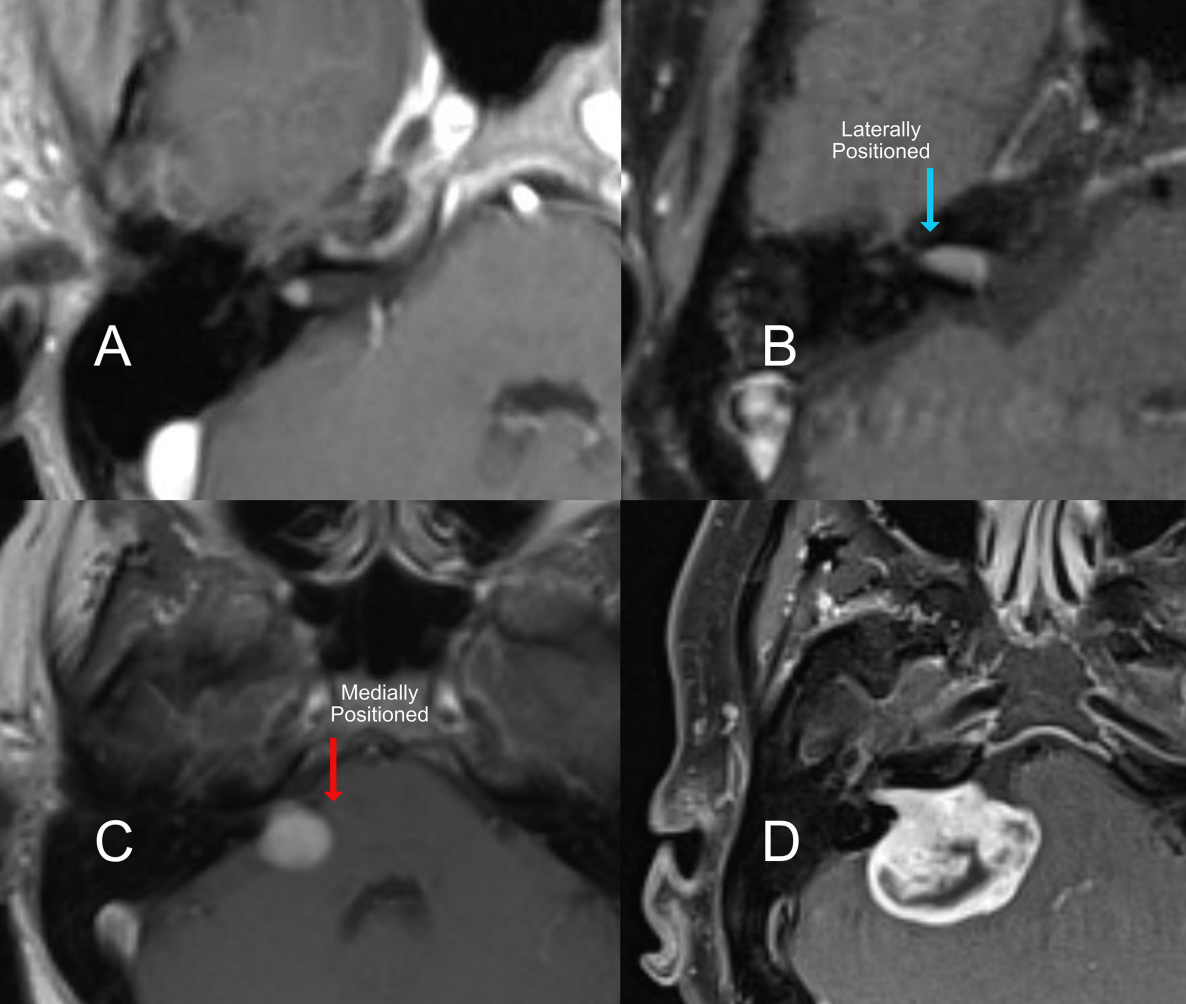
T1 Contrast MRI of an acoustic neuroma with different locations relative to internal auditory canal. (A) a very small intracanalicular tumor, (B) a laterally positioned tumor filling the auditory canal, (C) medium tumor medially positioned to the internal acoustic canal, and a large tumor (D) with brainstem compression.
